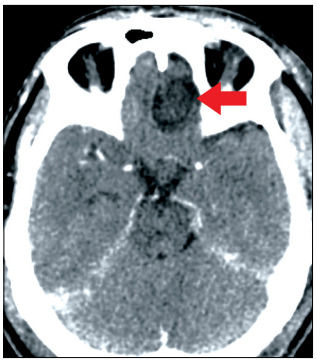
Brain computed tomography showing Simpson II resection of olfactory groove meningioma.
